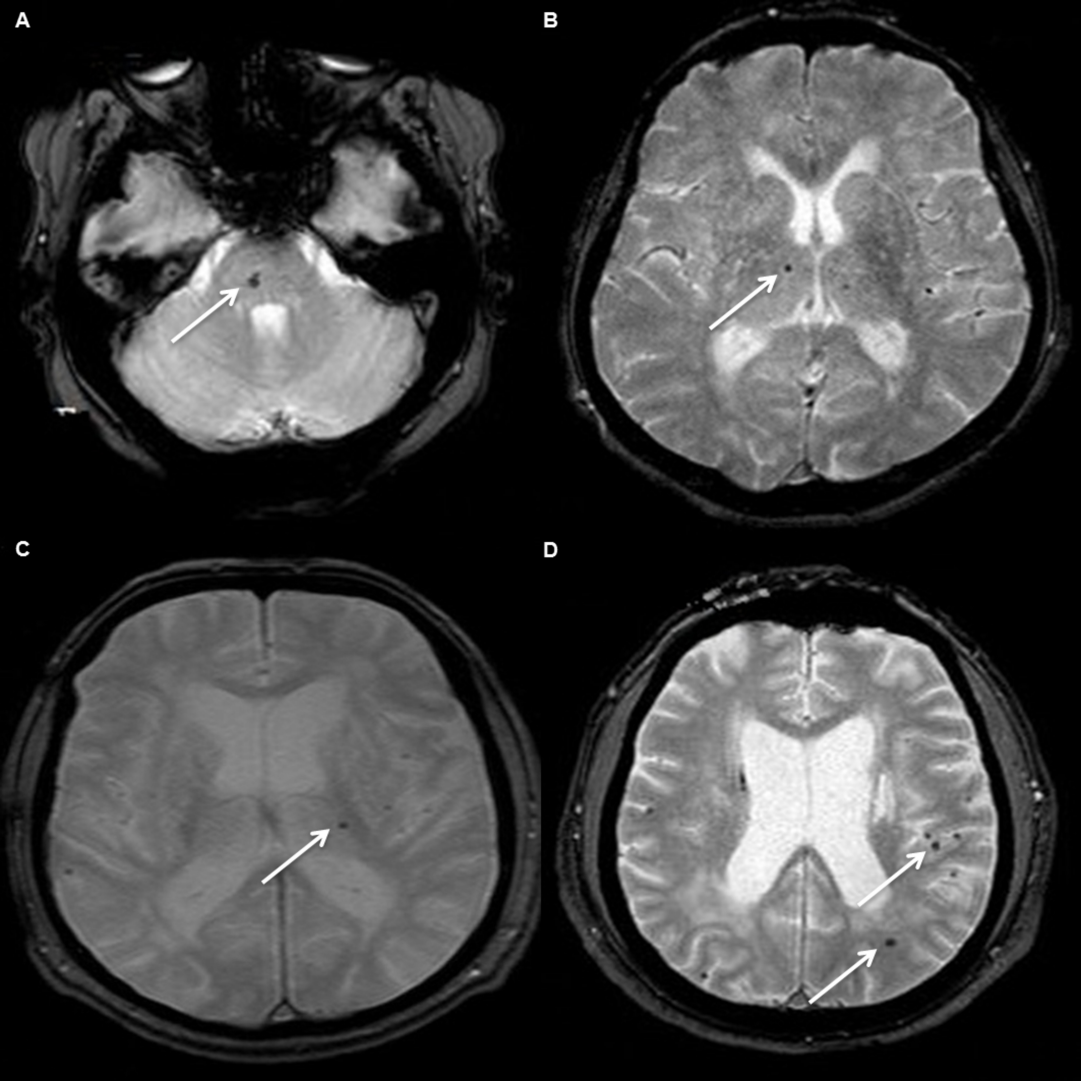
Examples of different locations of cerebral microbleeds in our sample of CADASIL patients. T2*-weighted magnetic resonance images showing cerebral microbleeds (white arrows) in infratentorial (A), deep subcortical (B, C) and lobar locations (D).
